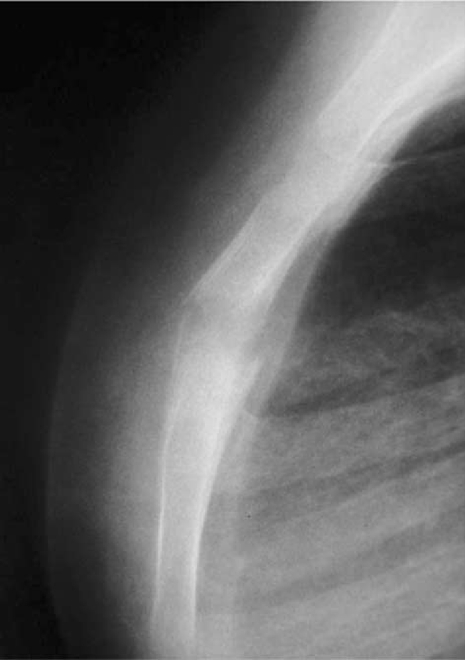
A lateral radiograph of the sternum showed an ill-defined osteolytic lesion in the sternum. Periosteal reactions around the lesion were also observed.
